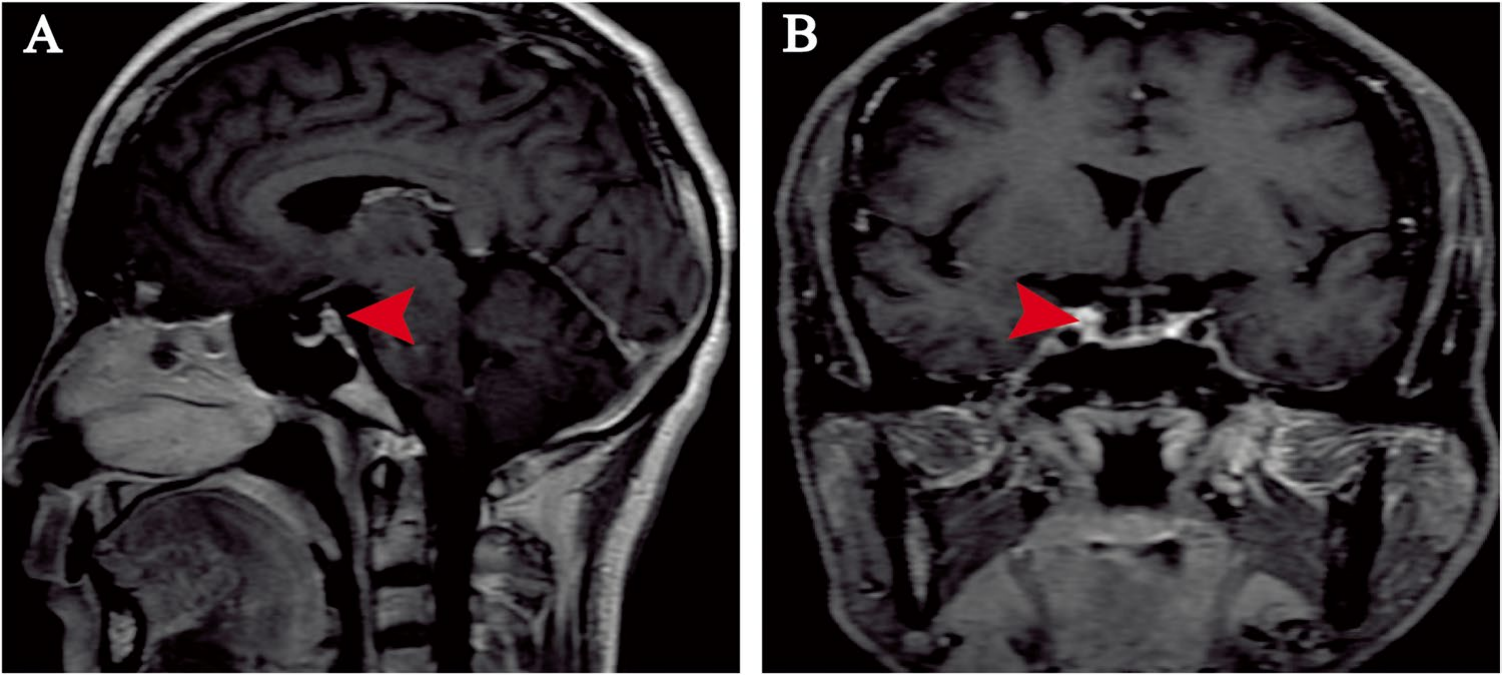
Magnetic resonance imaging (MRI) revealed a partially empty sella (arrow). A. Sagittal T1W. B. Coronal T1W
